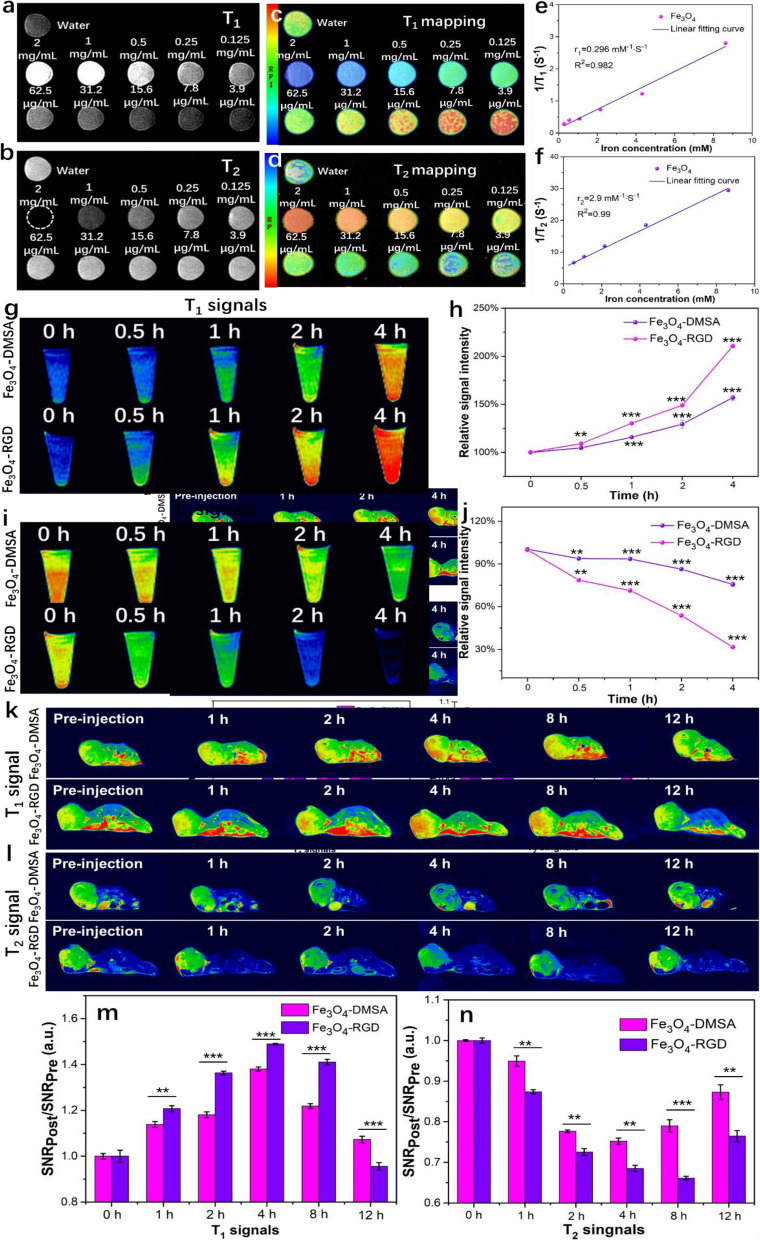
T_1_/T_2_ dual-mode MRI of Fe_3_O_4_-DMSA and Fe_3_O_4_-RGD nanoclusters both in vitro and vivo. (a) T_1_-weighted MR images, (c) T_1_ mapping and (e) T_1_ relaxation time of Fe_3_O_4_-DMSA nanoclusters at various iron concentrations. (b) T_2_-weighted MR images, (d) T_2_ mapping and (f) T_2_ relaxation time of Fe_3_O_4_-DMSA nanoclusters at various iron concentrations. (g) T_1_ MRI images of 4T1 cells cultured with Fe_3_O_4_-DMSA and Fe_3_O_4_-RGD nanoclusters and (h) the corresponding signal intensity statistics. (i) T_2_ MRI images of 4T1 cells cultured with Fe_3_O_4_-DMSA and Fe_3_O_4_-RGD nanoclusters and (j) the corresponding signal intensity statistics. (k) T_1_ MRI images of tumors in vivo at different times after the injection of Fe_3_O_4_-DMSA and Fe_3_O_4_-RGD nanoclusters. (l) T_2_ MRI images of tumors in vivo at different times after the injection of Fe_3_O_4_-DMSA and Fe_3_O_4_-RGD nanoclusters. (m) T_1_- and (n) T_2_-weighted MRI signal intensity statistics at different times.
